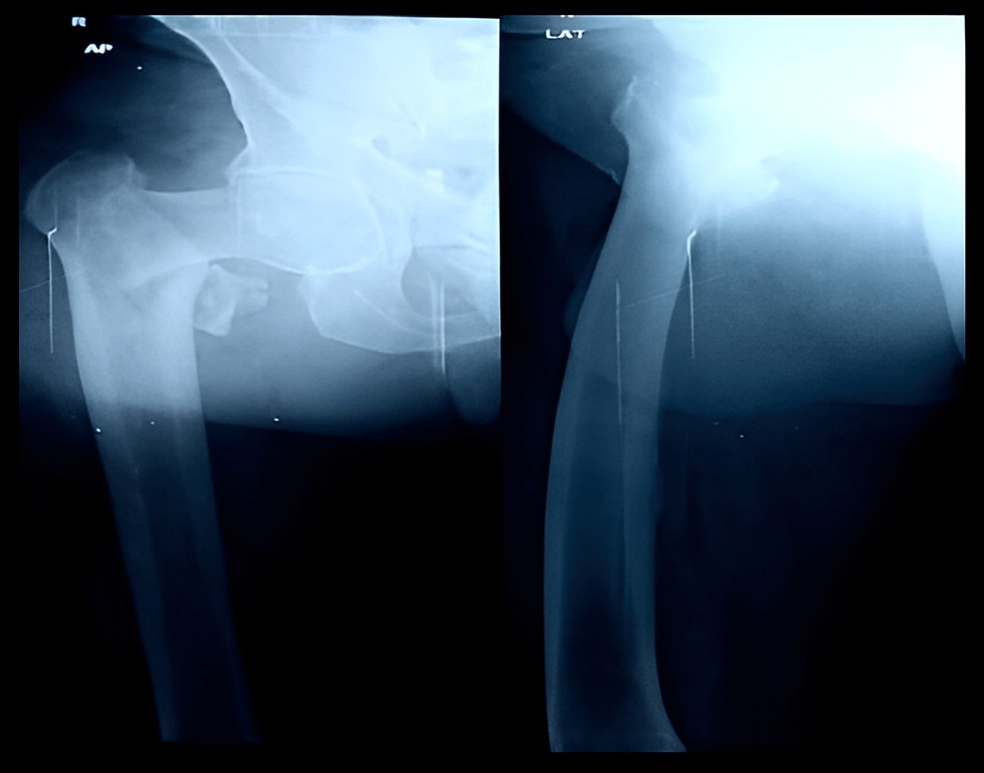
Anteroposterior X-ray view of a 70-year-old male showing an unstable intertrochanteric fracture of AO/OTA type 31-A2.3 of the right side AO/OTA type: Association for Osteosynthesis/Orthopaedics Trauma Association
